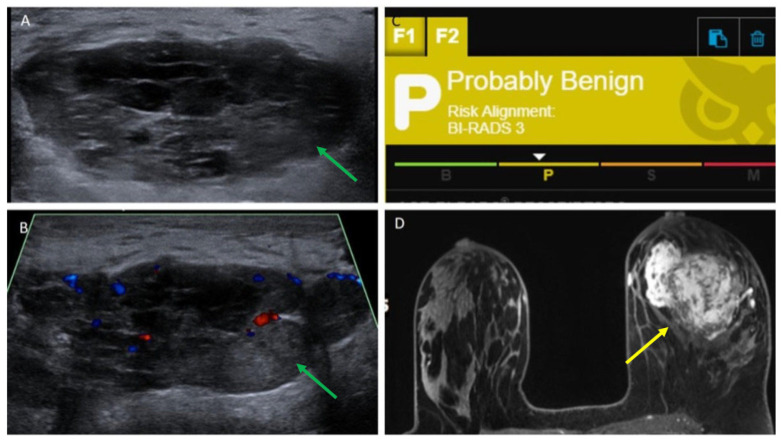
Patient 16 in Table 1 and Table 2, a 29-year-old woman with new palpable lump in the left breast. (A,B) Ultrasound targeted to the palpable lump showed a 5.5 cm oval, circumscribed, parallel hypoechoic mass (shown by green arrows) with mixed solid and cystic components, as well as internal vascularity. (C) AI DS assessment of probably benign was obtained. (D) Contrast-enhanced MRI showed a corresponding lobulated, irregular mass (yellow arrow) with heterogenous enhancement. Ultrasound-guided biopsy yielded angiosarcoma. The lack of color Doppler evaluation by AI DS may have contributed to the incorrect “probably benign” assessment.
